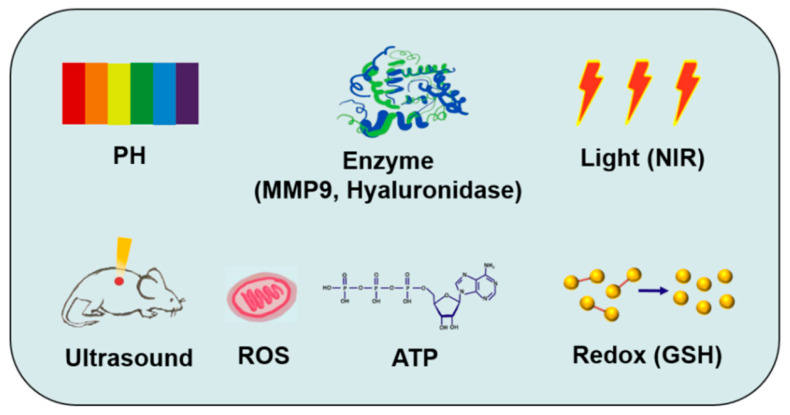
The main stimuli-responsive strategies of CPP-based macromolecular drug delivery. This figure lists the main stimulus factors used in stimuli-responsive strategies of CPP-based delivery systems in recent years, including pH, enzyme, light, ultrasound, ROS, ATP, and redox.
